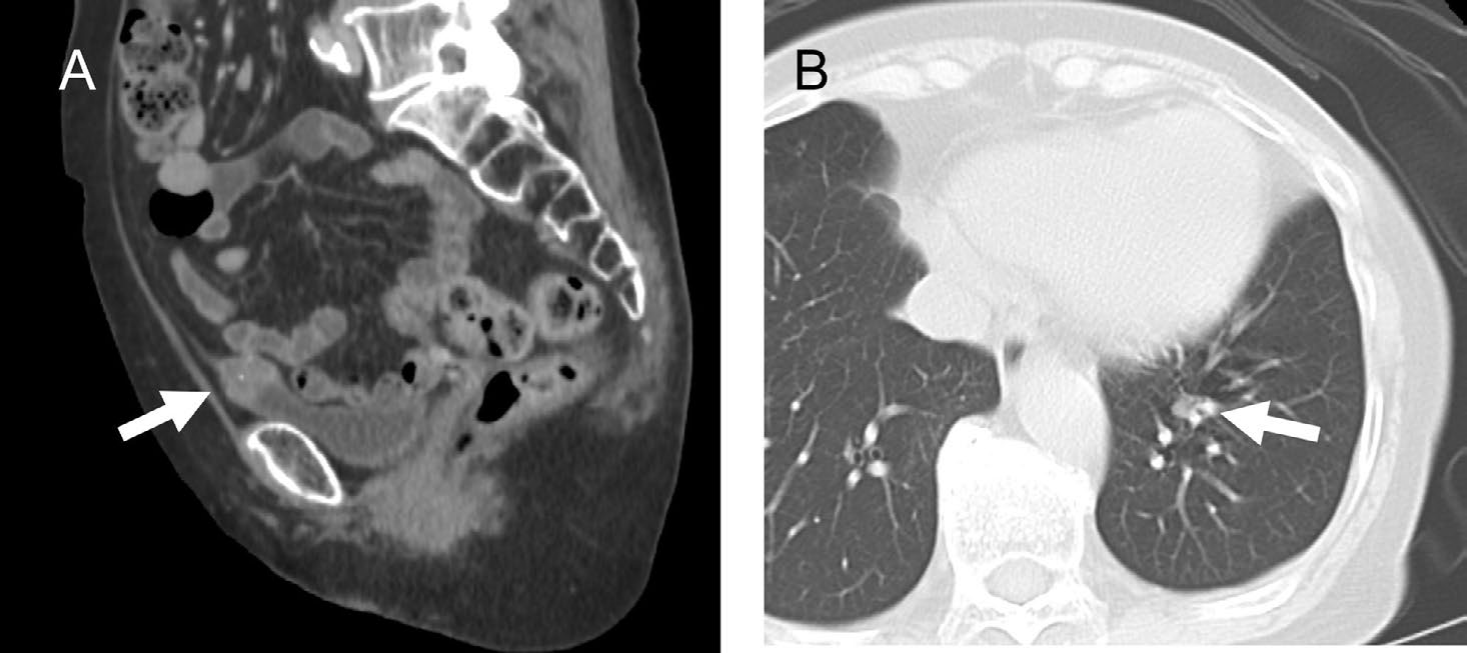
Computed tomographic image showing (A) a mass in dome of the bladder and (B) a nodule in the left lung.
